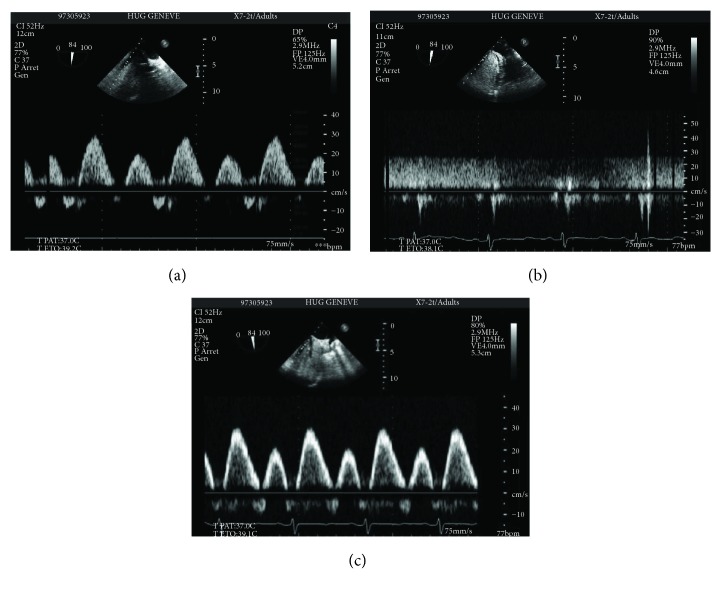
Hepatic blood flow at the middle hepatic vein measured by transesophageal echocardiography. (a) Normal triphasic Doppler signal of HBF from the MHV at time 2 (end of CRS, before abdominal filling). (b) Nonpulsatile Doppler signal at time 3 (end of HIPEC, before abdominal emptying). (c) After emptying abdominal cavity, at time 4 (end of procedure, after abdominal emptying and removal of abdominal drainage), HBF returns to normal Doppler signal waveform. HBF: hepatic blood flow; MHV: middle hepatic vein.
